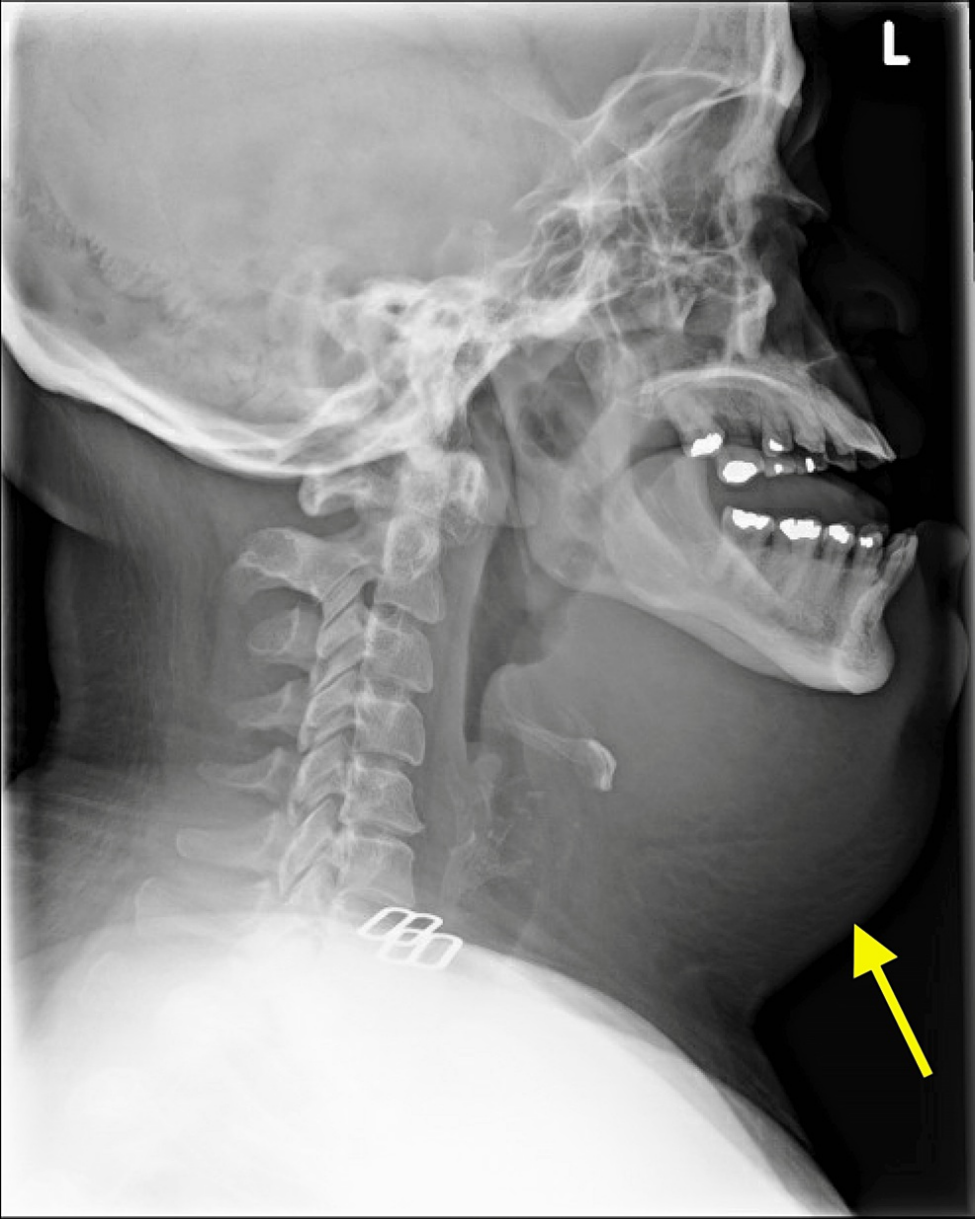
Lateral flat plate radiograph demonstrating submandibular soft tissue swelling (arrow)
